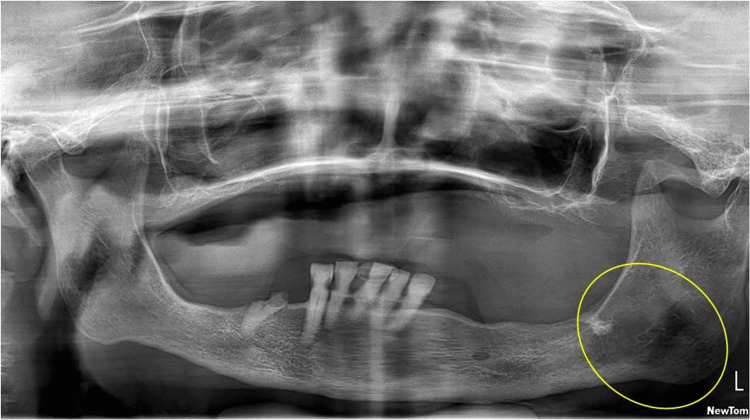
Panoramic radiograph (orthopantomography) showing radiolucent and radiopaque changes in the left mandibular body and angle. A well-defined radiopaque structure, consistent with a retained or impacted root undergoing osseointegration, is observed in the region of the left posterior mandible (highlighted in the yellow circle). Adjacent to this, an ill-defined radiolucent area extends through the posterior mandibular body and angle, associated with cortical thinning and partial involvement of the inferior alveolar canal, suggesting the presence of an infiltrative lesion.
